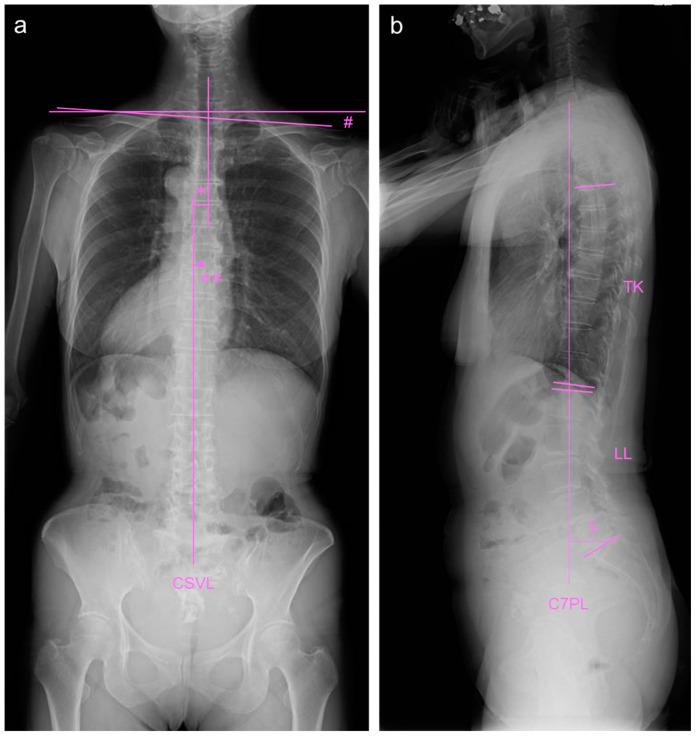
Standing whole-spine radiographs after six months daily physical exercises. (a) Antero-posterior whole-spine radiograph. # indicates clavicle angle, * indicates C7-CSVL measurement, and  indicates apex-CSVL measurement. (b) Lateral whole-spine radiograph. C7PL: C7 plum line, TK: thoracic kyphosis, LL: lumbar lordosis, sagittal vertical axis: SVA. § indicates sagittal vertical axis (SVA).
